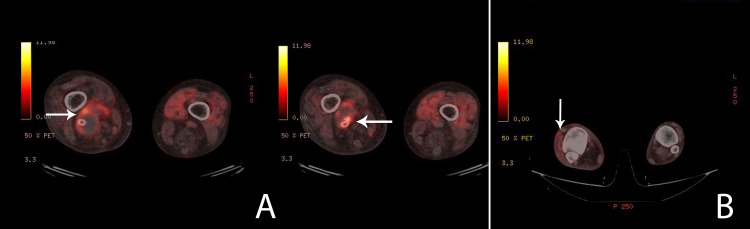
PET/CT showing increased metabolic activity. A: Increased metabolic activity in the aneurysm sac.
